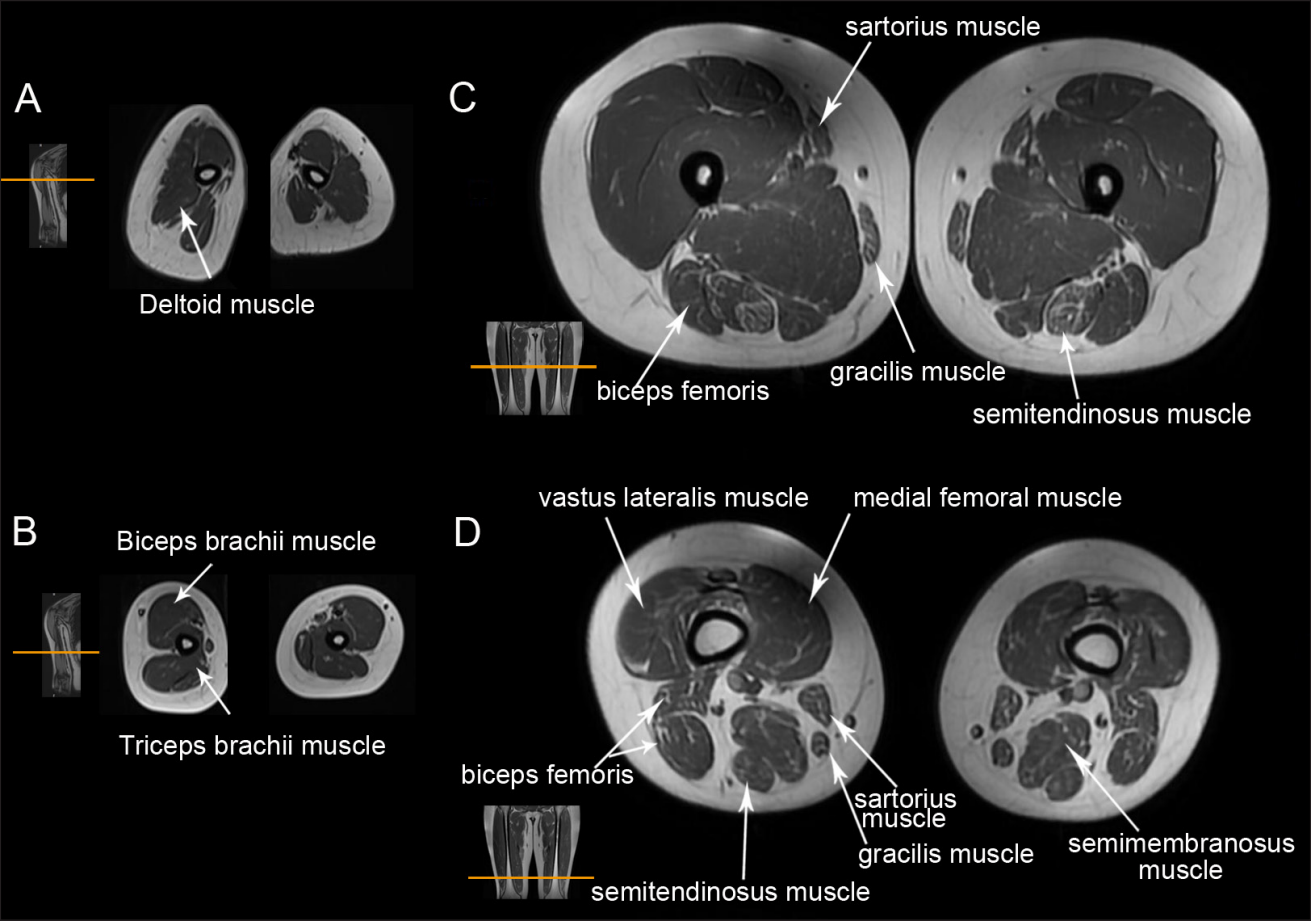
T1-weighted transverse magnetic resonance images of the patient’s arms (A–B) and thighs (C–D). Mild fatty infiltration of the deltoid muscle and triceps brachii was observed in the upper arms. Mild-to-moderate fatty infiltration was observed in the biceps femoris, quadriceps femoris, sartorius, semitendinosus, semimembranosus, and gracilis muscles.
